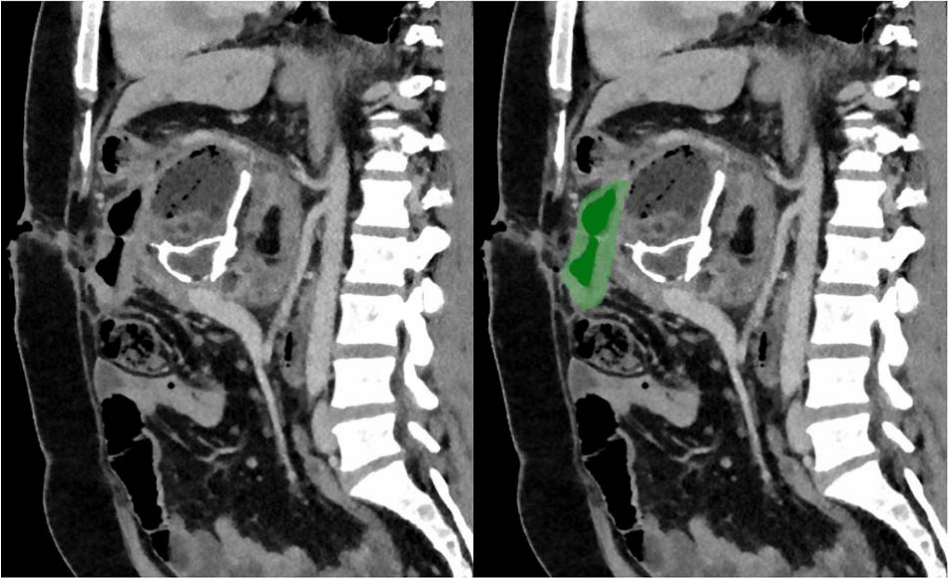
Sagittal section of post-operative CT with portal venous contrast demonstrating cystojejunustomy, highlighted in green, anastomosed to the origin of the fistula.
